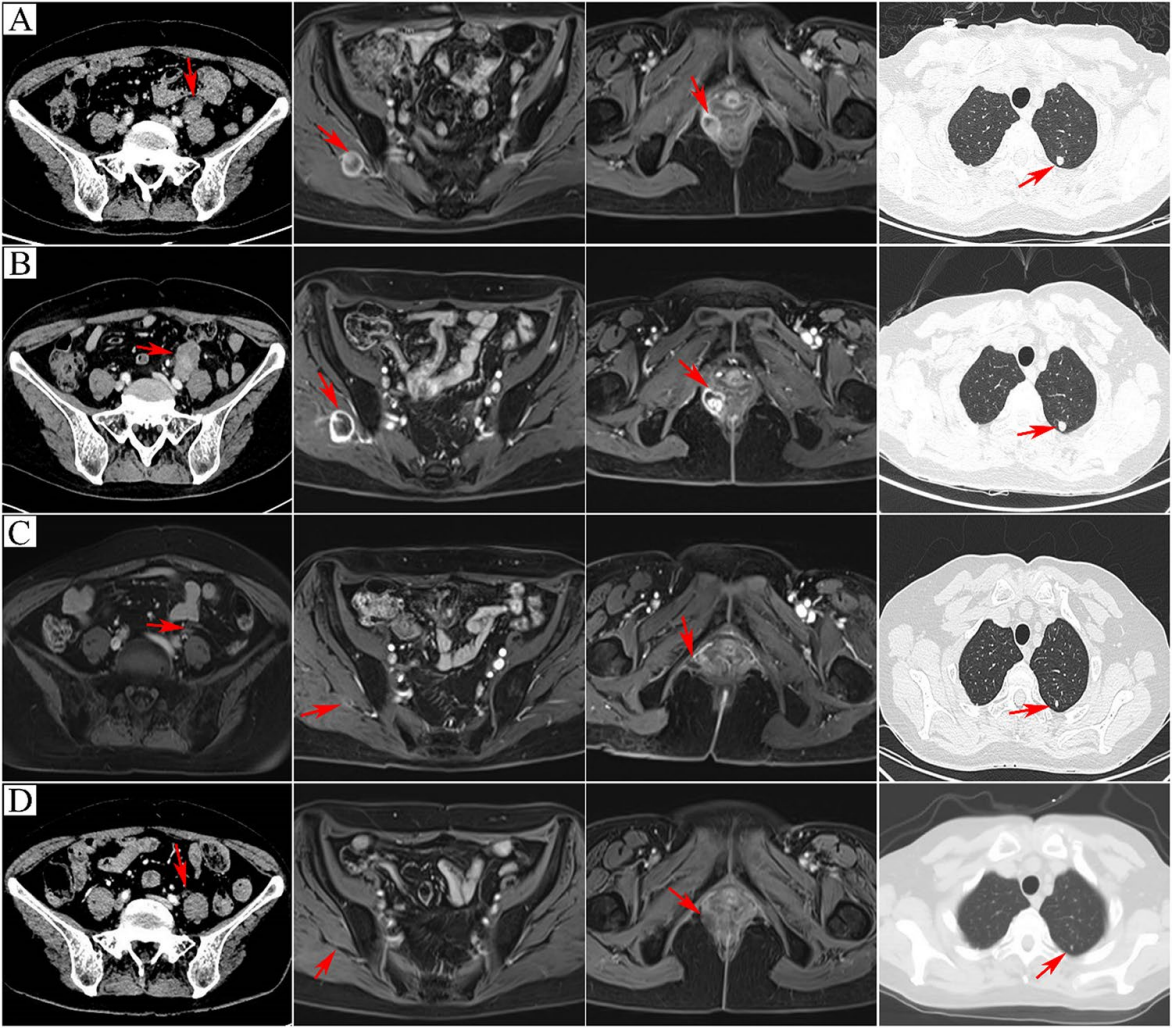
Abdominal MRI and lung CT scans. A Postoperative imaging revealed metastatic foci in para-iliac vessels, right gluteus maximus space nodule, right levator ani muscle, and left lung without any drug intervention. B After two cycles of sintilimab combined with anlotinib, the metastatic tumor either progressed or remained stable. C Following five cycles of cadonilimab combined with anlotinib, the metastatic tumor of the metastatic focus was significantly smaller and improved compared to previous scans. D After seven cycles of treatment with cadonilimab combined with anlotinib, the metastatic tumor essentially returned to normal compared to the pre-treatment state
